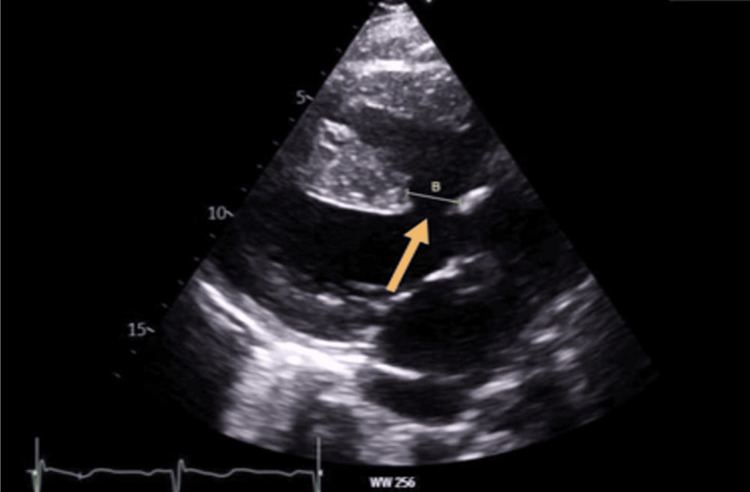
Yellow arrow depicts ventricular septal defect (VSD) measuring B=2cm in parasternal long axis ECHO view (PLAX). Yellow arrow shows similar finding in apical 4 chamber echocardiographic view
